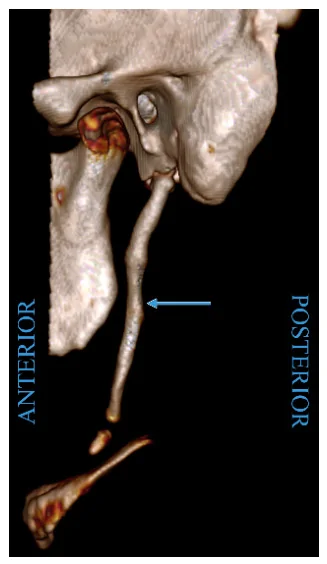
Elongated left styloid process (arrow). Left side, lateral view. Computed tomography. Three-dimensional volume rendering.
